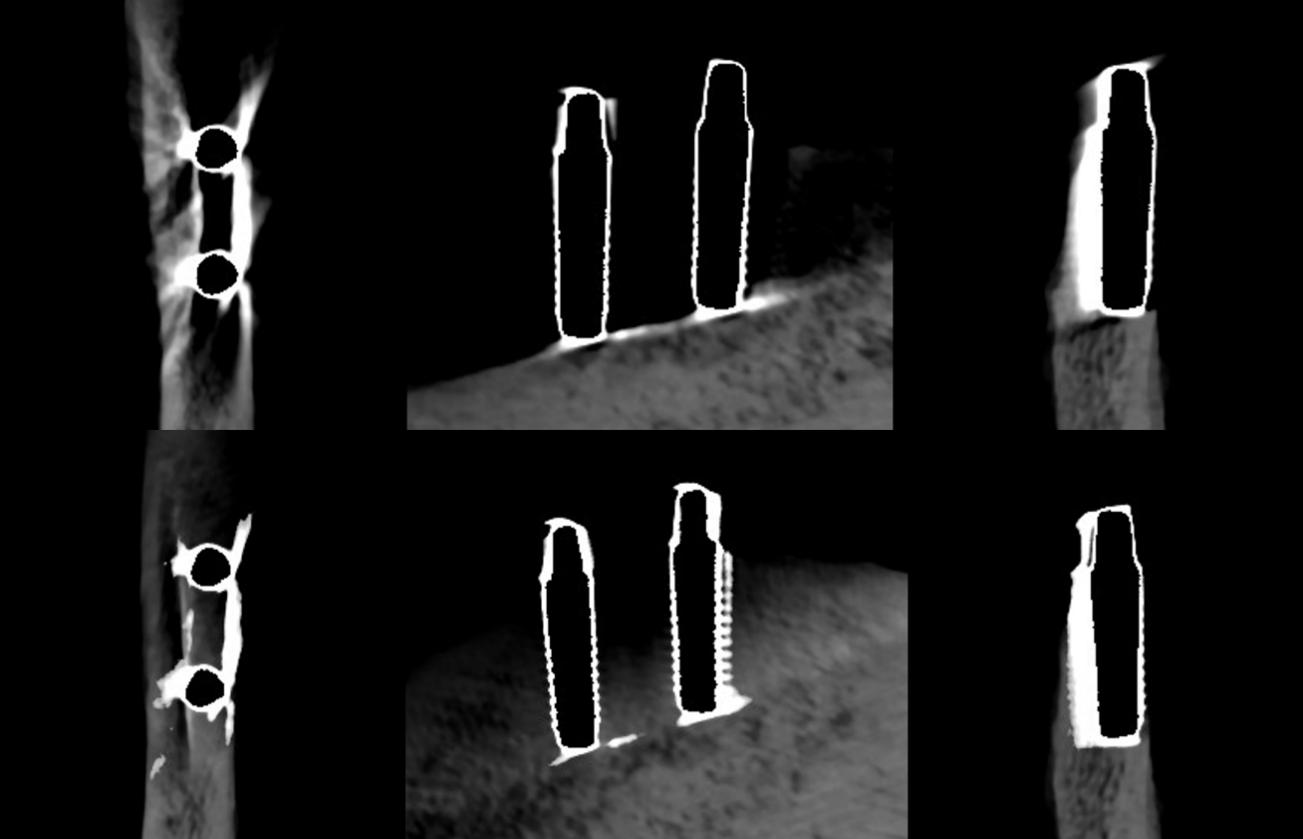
Axial, sagittal, and coronal slices of the CBCT image of the zirconia implants with the imaging parameters 3. Above no ARA and below ARA 3. The defect cannot be seen and the bone structure between the implants is not smooth. In addition, the zirconia implants are seen centrally radiolucent with a radiopaque rim with or without ARA. ARA, artifact reduction algorithm; CBCT, cone beam CT.
